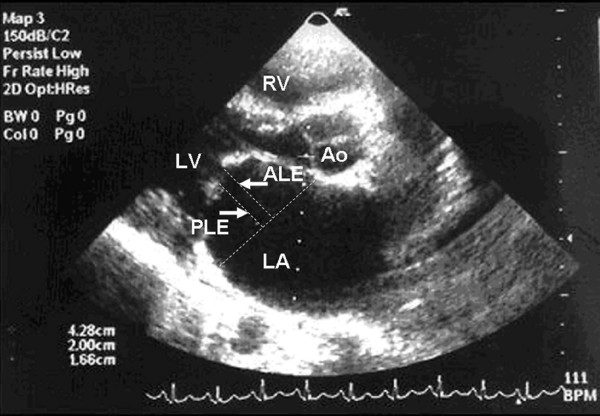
Anterior and posterior leaflets excursion measured in parasternal long axis view. RV, right ventricle; LV, left ventricle; LA, left atrium; Ao, aorta; ALE, anterior leaflet excursion; PLE, posterior leaflet excursion.
